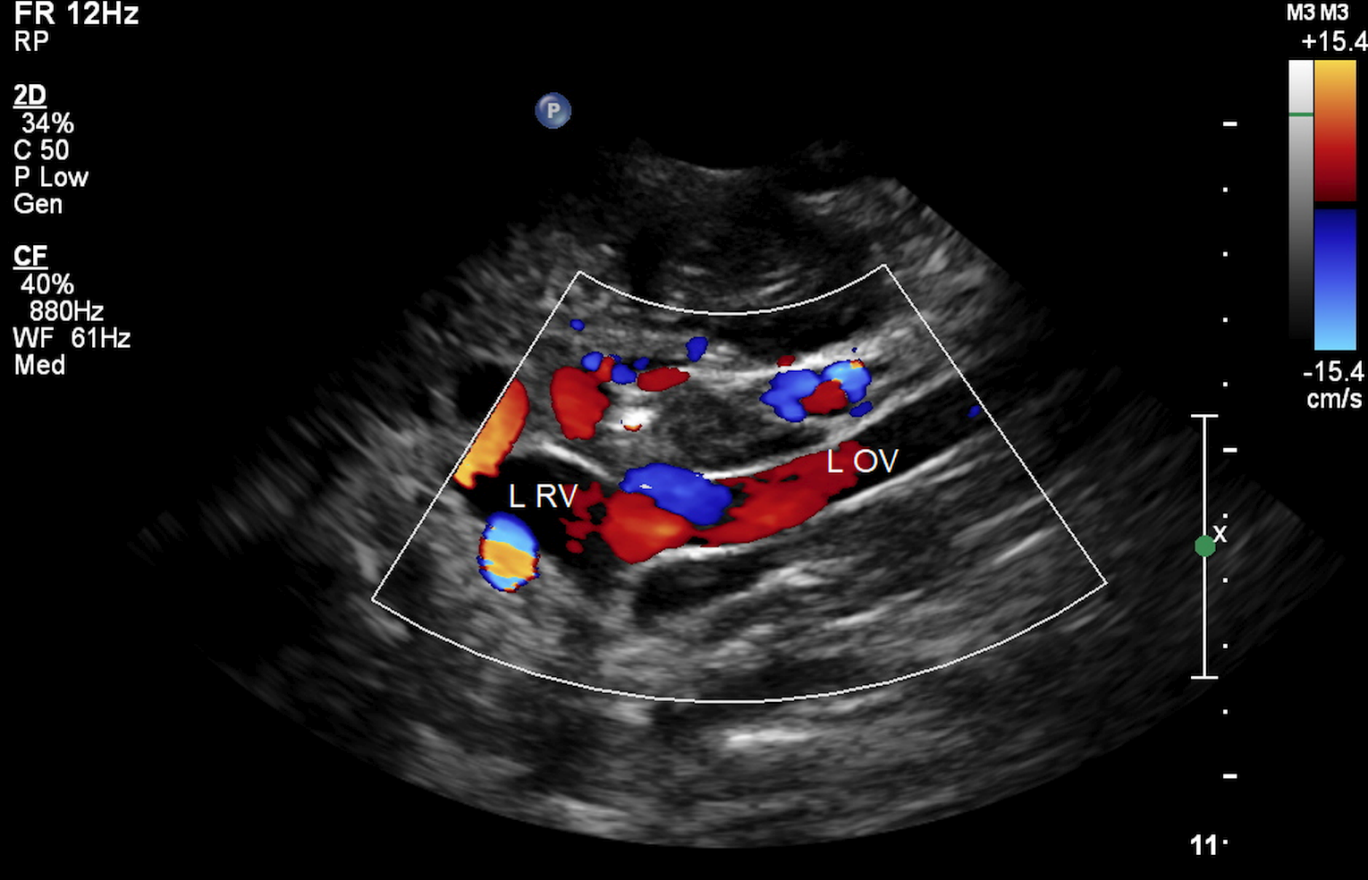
Ovarian vein reflux on color Doppler imaging. L RV, left renal vein; L OV, left ovarian vein.
